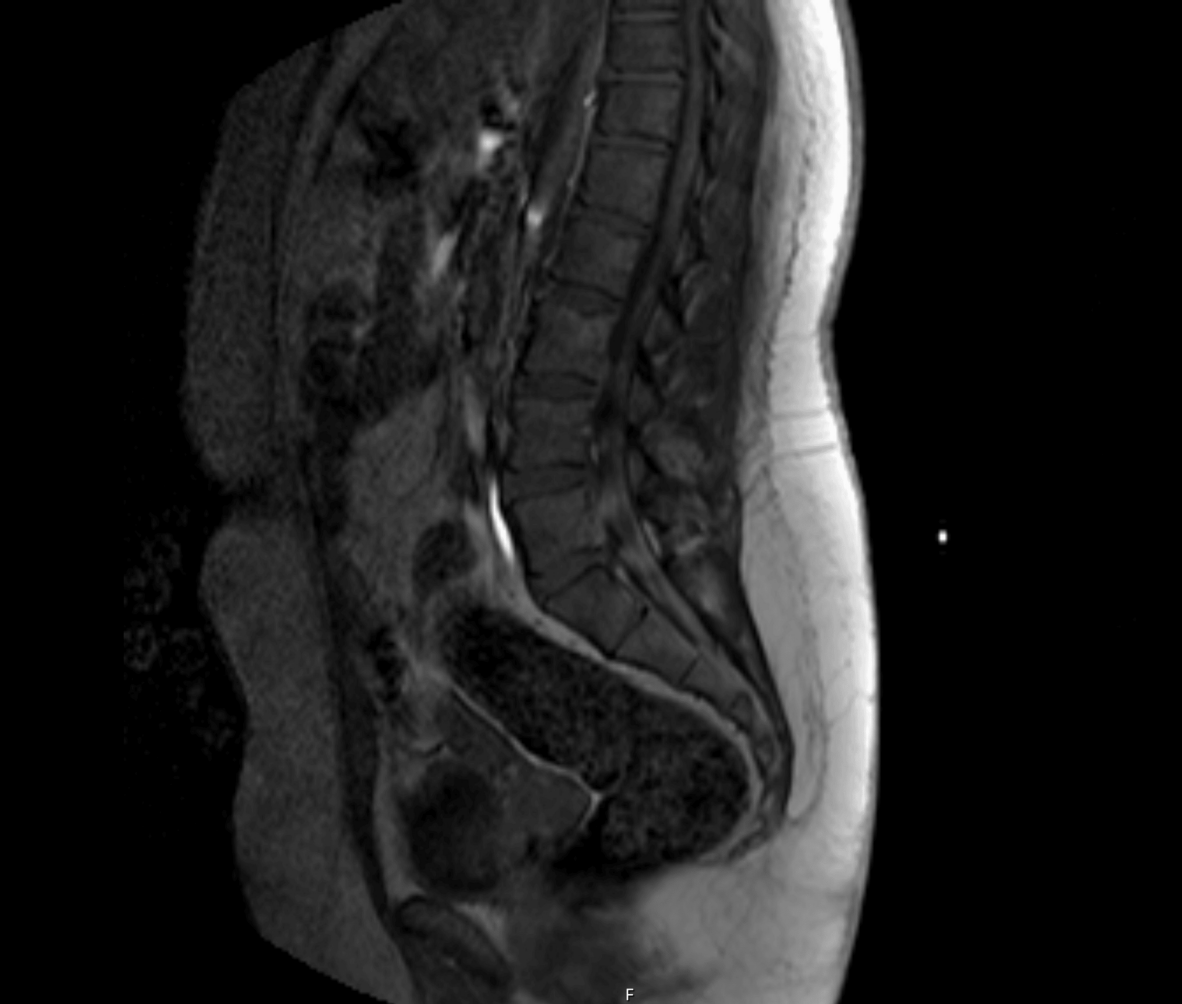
MRI of lumbar spine MRI of lumbar spine showing the normal cord signal and absence of a compressive lesion.
